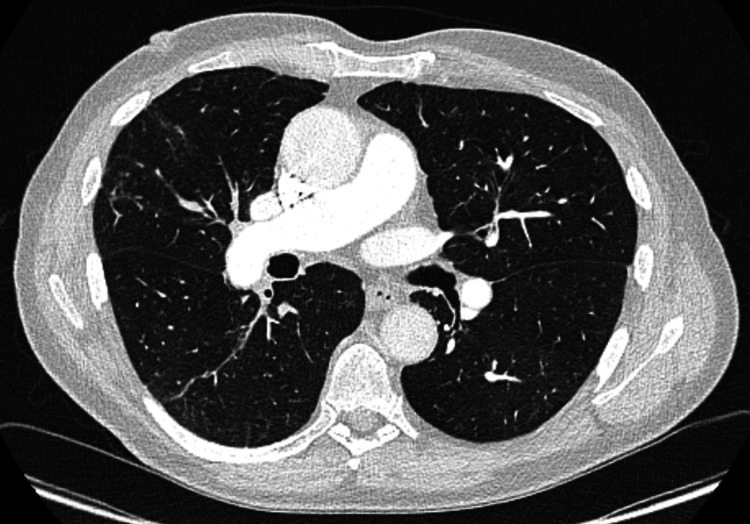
CT pulmonary angiogram (CTPA) eight months later showing complete resolution of the cavitating lesion
